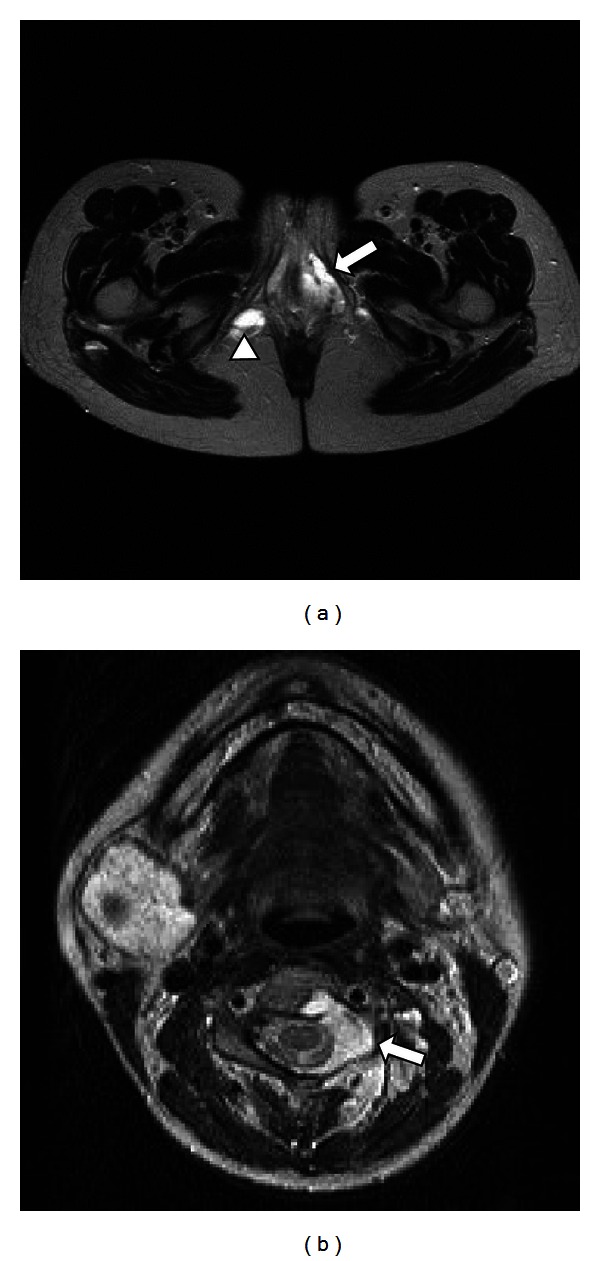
T2-weighted pelvic and brain magnetic resonance imaging (MRI). (a) Pelvic MRI demonstrating venous malformations in the left vulva (arrow) and right levator ani (arrow head). (b) Brain MRI exhibiting spinal epidural venous malformations (arrow).
